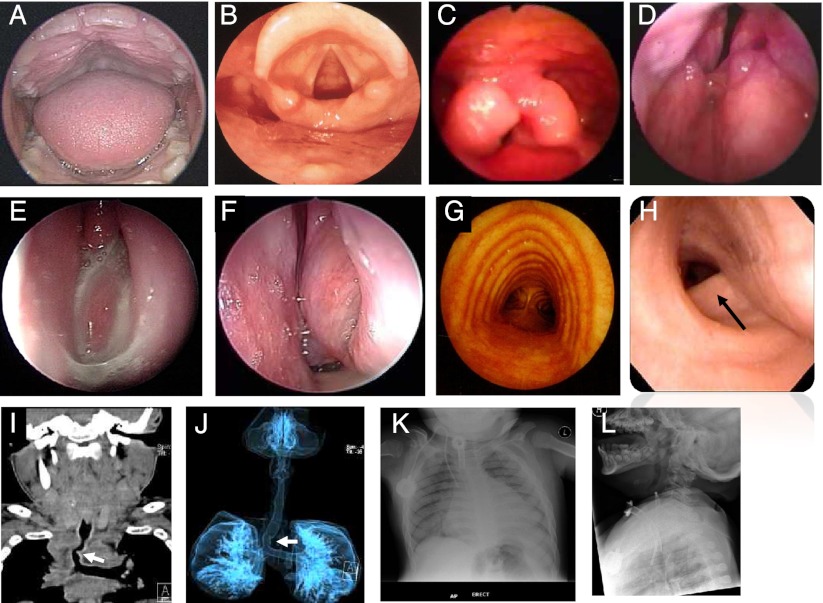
a Oropharyngeal image from a patient with mucopolysaccharidosis (MPS) II showing macroglossia and gingival hyperplasia; b normal lyaryngoscopic image; c-d laryngoscopic images from a patient with MPS II showing a thick laryngeal wall, tonsillar hyperplasia, and redundant arytenoid mucosa prolapse into laryngeal inlet; e-f nasal endoscopic images showing inflammation and abnormal mucous secretion in a patient with MPS VI; g normal bronchoscopic image (Canani et al 2003); h-j bronchoscopic image and CT scans from a patient with MPS IV showing tracheal deformity and narrowing; k-l typical frontal and lateral plain X-rays of the chest of a child with MPS II showing skeletal abnormalities and the presence of a tracheostomy and possibly infective changes in the lungs (hazy shadow in the right lower zone of the frontal image)
